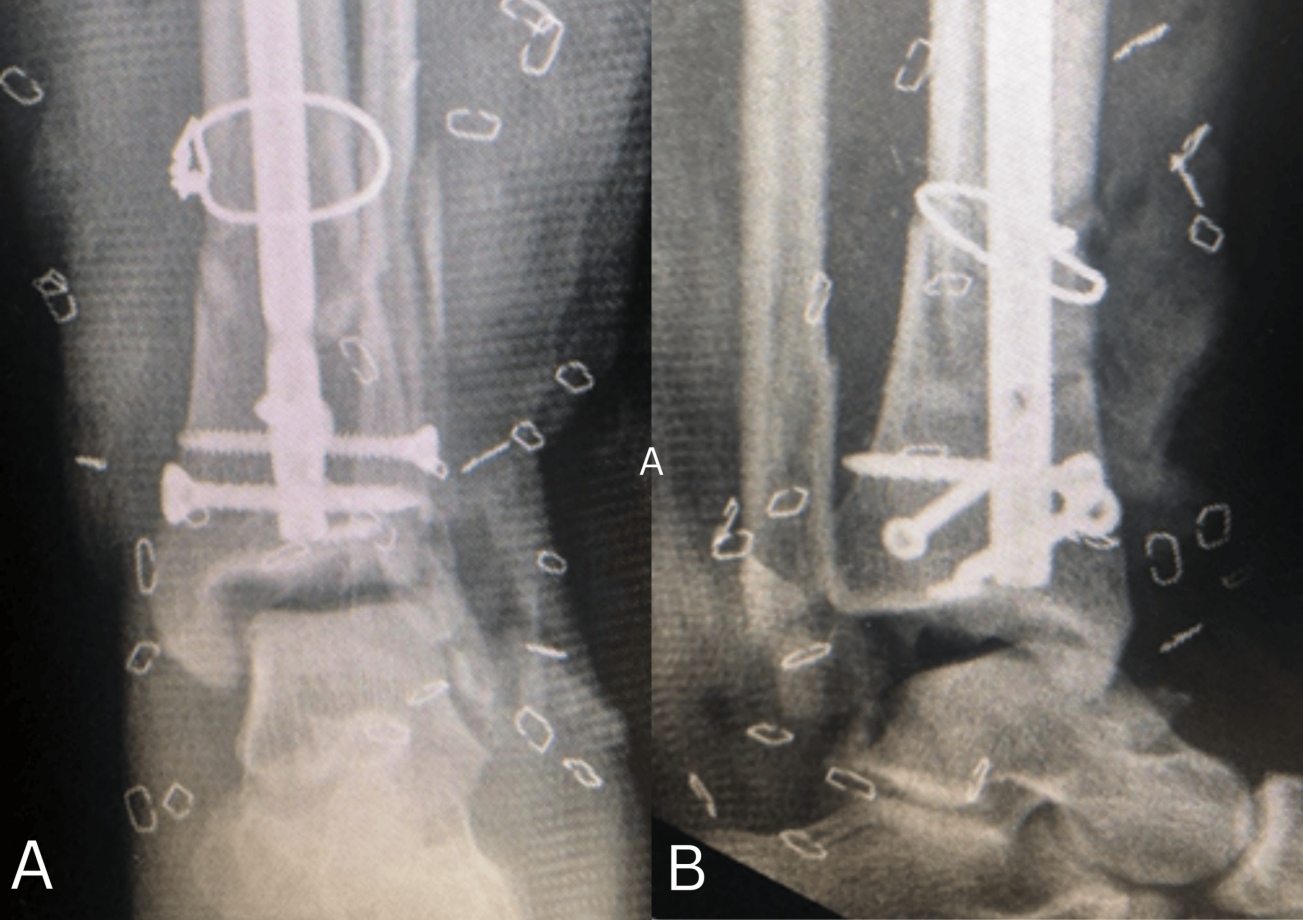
Immediate postoperative radiographs A. Anteroposterior view demonstrating an intramedullary tibial nail with distal interlocking screws and cerclage wiring, with maintained alignment. B. Lateral view confirming appropriate hardware positioning and satisfactory sagittal alignment of the distal tibia and ankle.
